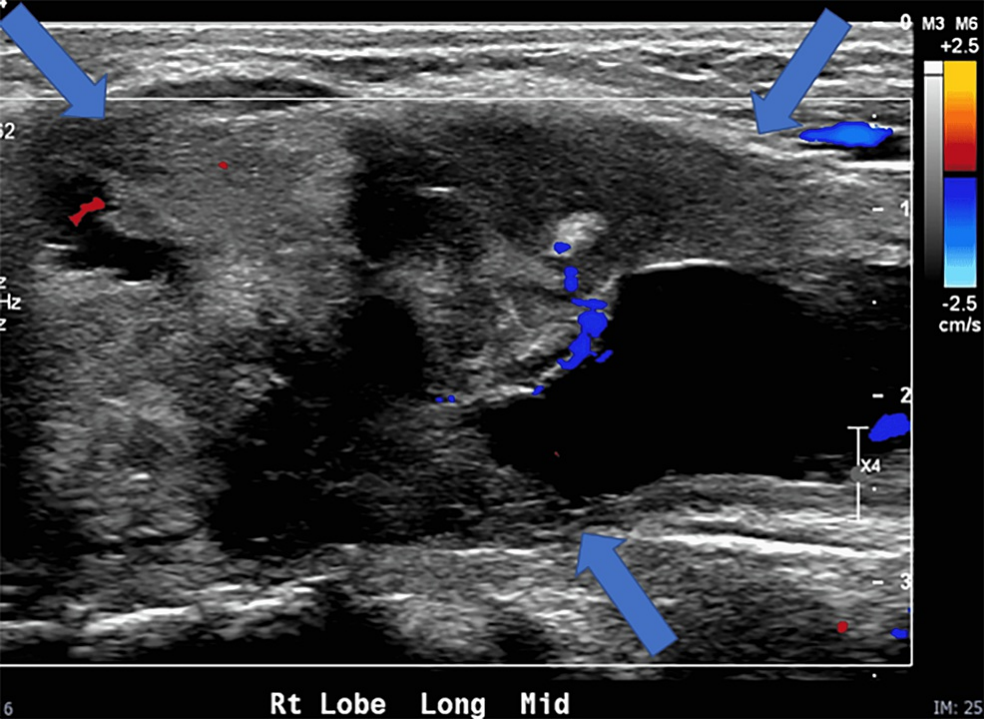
Longitudinal ultrasound image with color Doppler demonstrates the heterogeneous echotexture of the solid and cystic mass in the right lobe of the thyroid gland (blue arrows). Image courtesy: This image was provided by Dr. Laura L. Hayes from the radiology department at Nemours Children’s Health System.
